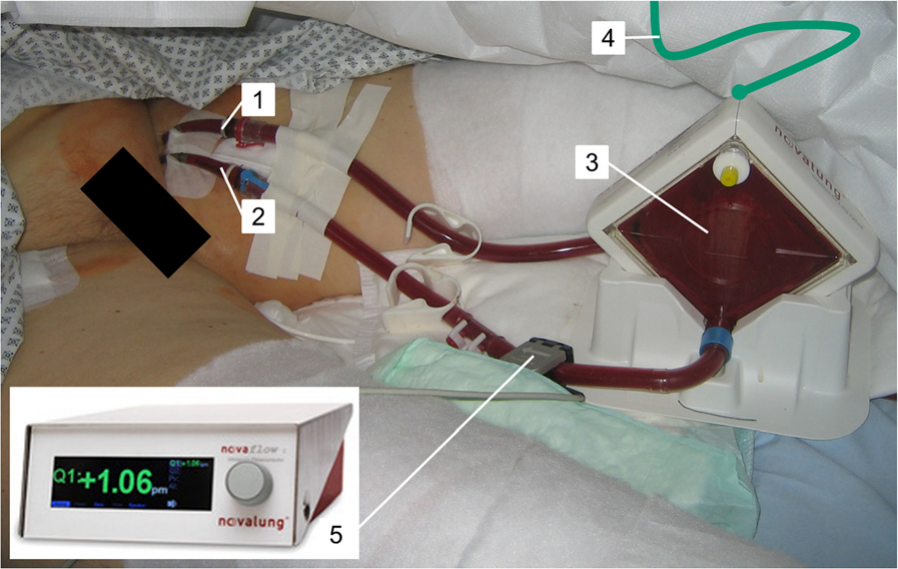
Clinical setup and components of the pumpless, arterio-venous ECCO_2_R circuit “interventional lung assist” (iLA®, Novalung GmbH, Heilbronn, Germany). The patient has given written consent for publication of this picture. 1 = arterial cannula, 2 = venous cannula, 3 = circuit including membrane for ECCO_2_R, 4 = sweep gas (O_2_), 5 = ultrasonic flow-meter
